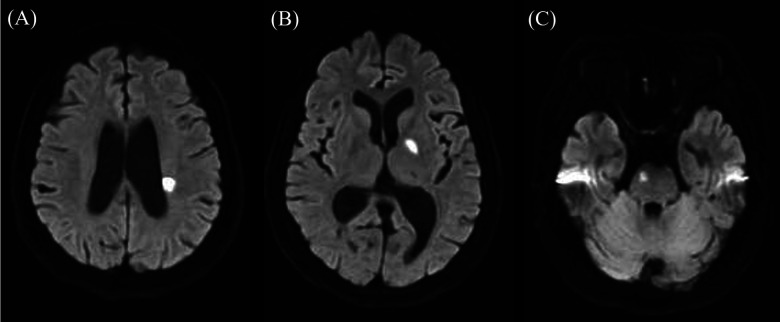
Diffusion-weighted brain magnetic resonance imaging (MRI) findings. (A,B) Diffusion-weighted imaging showing a hyperintense lesion extending about 20 mm from the left corona radiata to the basal ganglia. (C) Hyperintensity observed in the right pons. Image slice thickness: 5 mm.
